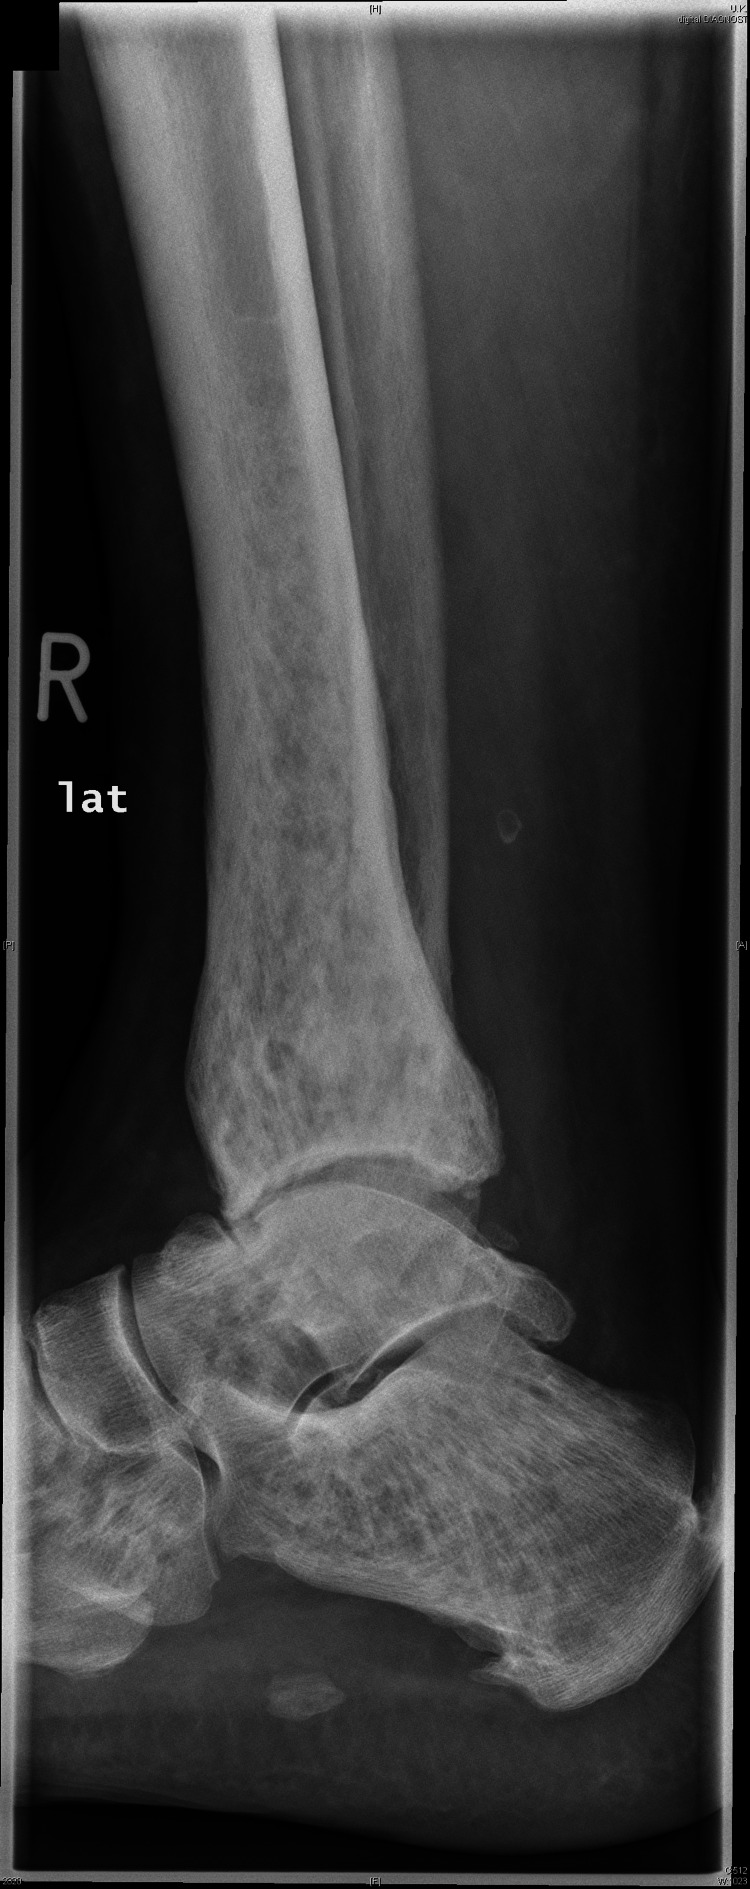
A lateral view of the right tibia, talus, and calcaneus in a conventional X-ray showed small, spotted, and partially confluent areas.
